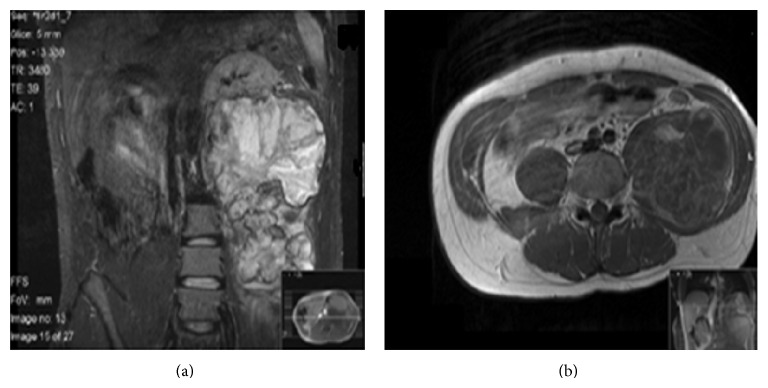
MRI scan (a) coronal view showing a large left lesion arising from the L3 vertebrae displacing the left kidney superiorly. (b) Axial view at the level of L3 depicting the lesion and its complete obliteration of the left psoas muscle.
